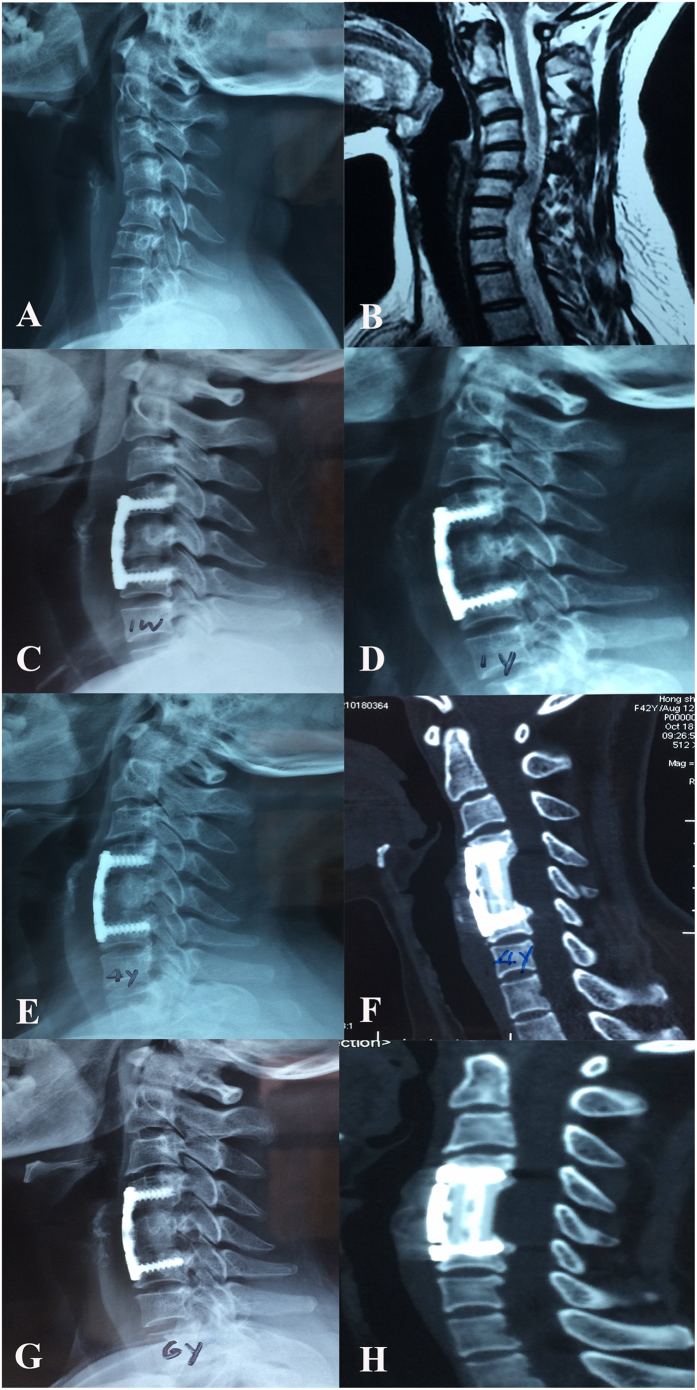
A 38-year-old woman who underwent 1-level corpectomy with a nano-hydroxyapatite/polyamide66 strut for cervical reconstruction. The preoperative cervical X-ray film (A) and MRI (B) revealed C5/6 disc herniation and segmental cervical kyphosis. The patient underwent a C5 corpectomy and fusion with an n-HA/PA66 strut (C). The strut and internal fixation were in position after 1 year of follow-up (D). The lateral X-ray film (E) and 3D-CT (F) scan indicated that the autogenous bone granules filling the strut had achieved bony fusion with adjacent endplates at the 4-year follow-up. The radiographic films revealed satisfying bony fusion with no obvious strut migration or subsidence at the 6-year follow-up ; no radiolucent gap occurred at the interface between the strut and the adjacent vertebrae (G,H).
